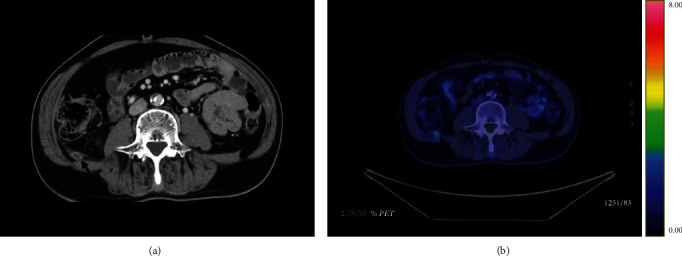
Appearance of a mass underneath in right side of the abdominal wall (arrow). (a) Contrast-enhanced computed tomography. (b) [18F]fluorodeoxyglucose- (FDG-) positron emission tomography-computed tomography shows increased FDG uptake with a maximum standardized uptake value of 3.2.
